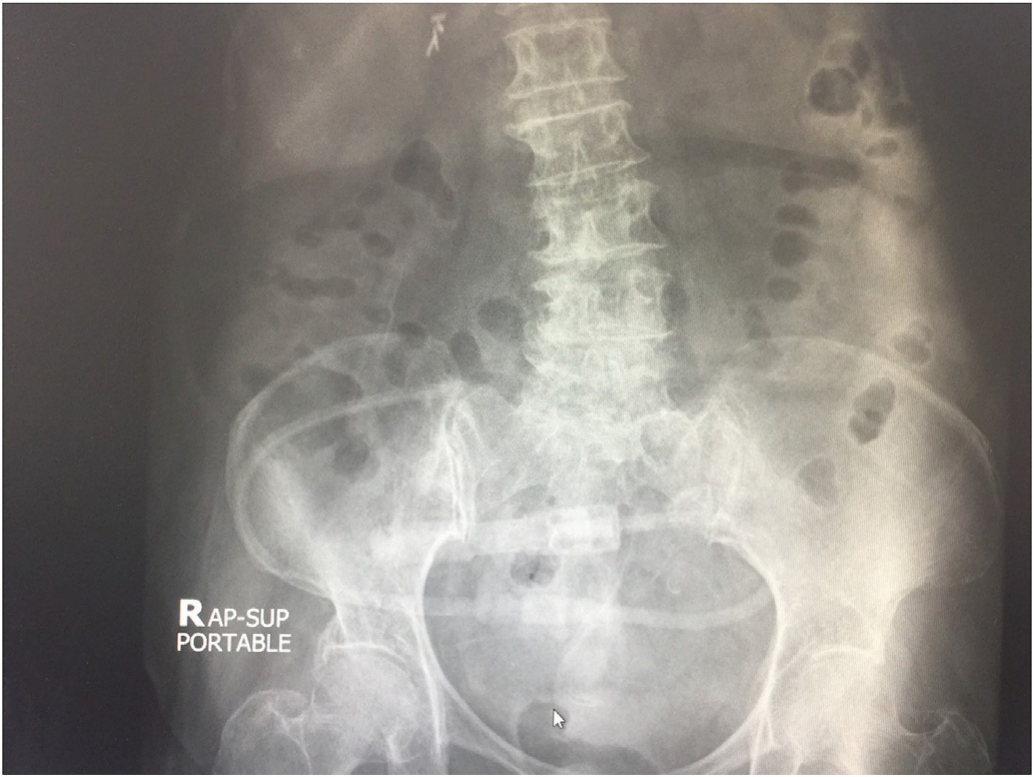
An abdominal X-ray showed an optimal position of the PD catheter.
